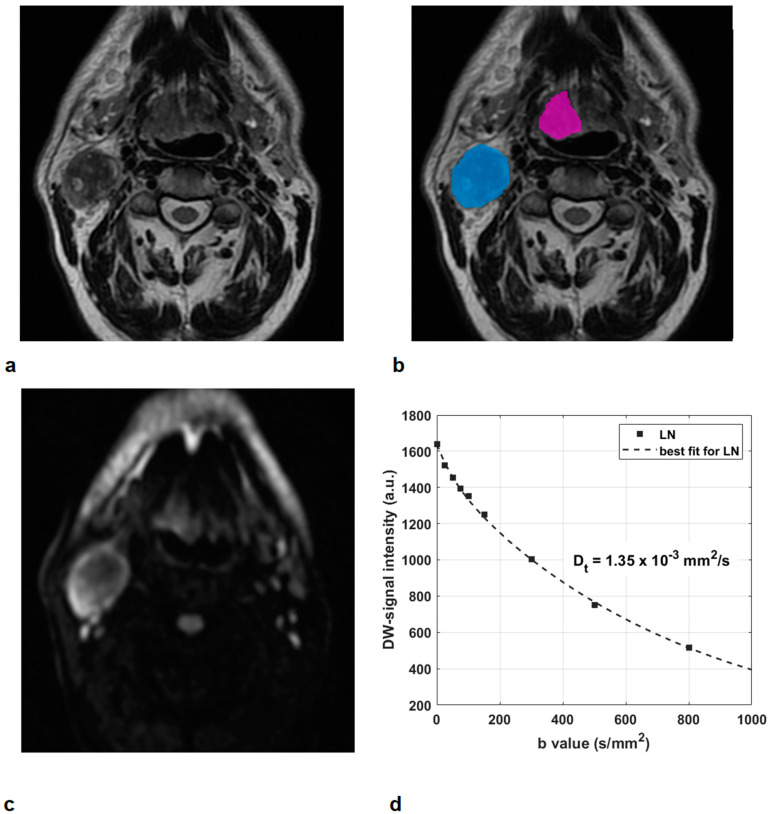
HPV-negative oropharyngeal squamous cell carcinoma of the base of the tongue in a 63-year-old moderate smoker and alcoholic man (Patient 62): T2-w images (a,b) show a primary tumor with an exophytic appearance and ulcerations (contour in magenta) and a large lymph node (LN) with extracapsular spread within IIa and IIb levels (contour in light blue). The corresponding DWI at b = 800 s/mm^2^ (c) and DW-signal intensity curve at increasing b values of the LN (d). A moderate restriction of water molecule mobility was observed in the LN, with ADC and D_t_ values of 1.52 and 1.35 × 10^−3^ mm^2^/s, respectively.
